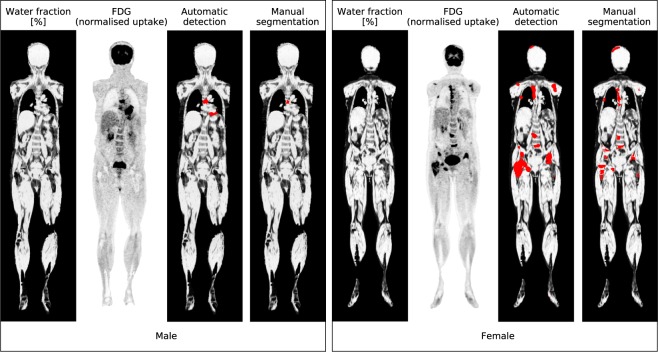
FDG anomaly detection task performed for the male (left) and female (right) subjects with suspected oesophageal cancer and lymphoma, respectively. For each subject, a representative coronal slice is shown of the registered water fraction and normalised FDG uptake images, and of the automatically detected and manually segmented tumours. Detected and segmented lesions are displayed in red and overlaid on the water fraction image. A false positive lesion can be seen for the male subject, likely due to increased motion artefacts in the heart.
